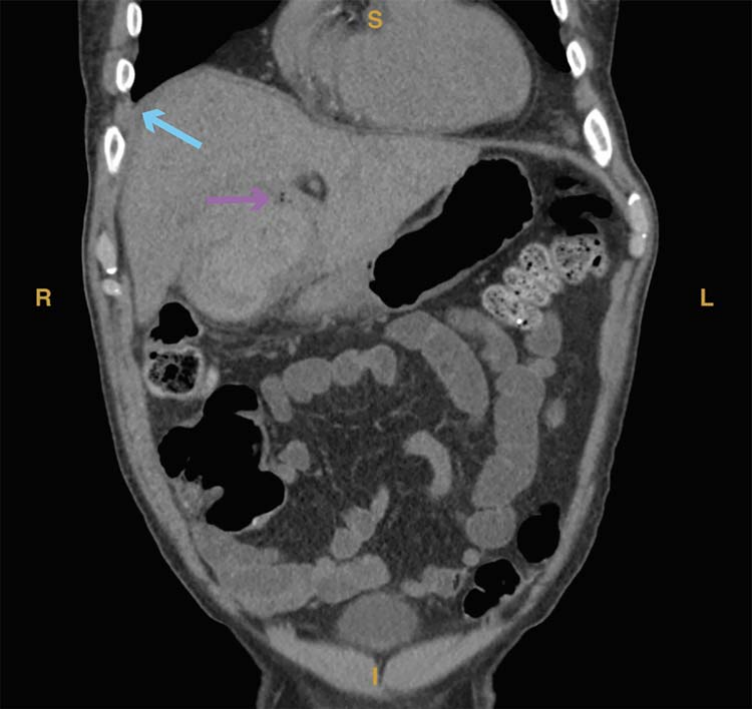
Computed tomography imaging from Case 2 showing air in the gallbladder wall (magenta arrow) as well as intraluminal fluid (cyan arrow) consistent with hemorrhage. Present in the figure, the letters S, L, I and R denote superior, left, inferior and right, respectively.
